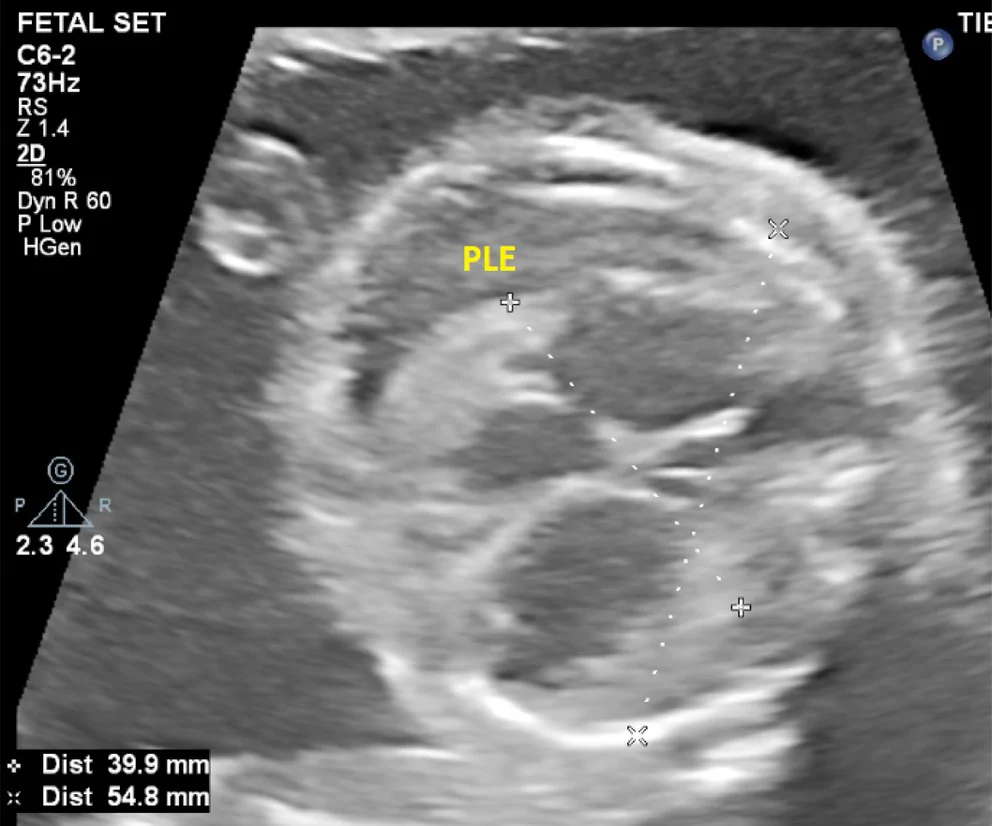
Follow‐up axial echocardiographic view obtained several weeks later demonstrates massive cardiomegaly, biventricular hypertrophy, and bilateral pleural effusions (PLE).
